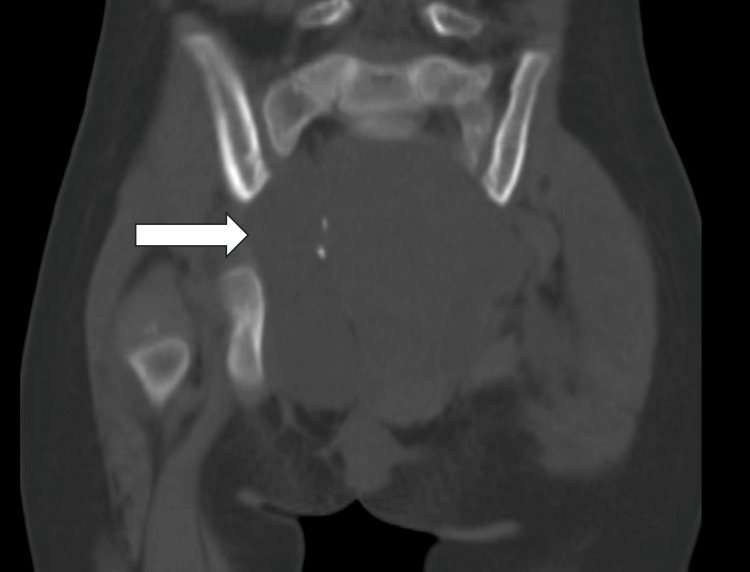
CT image. Complex solid lesion with few cystic components and a few calcific foci seen in the pelvis, in the pre-sacral region.
